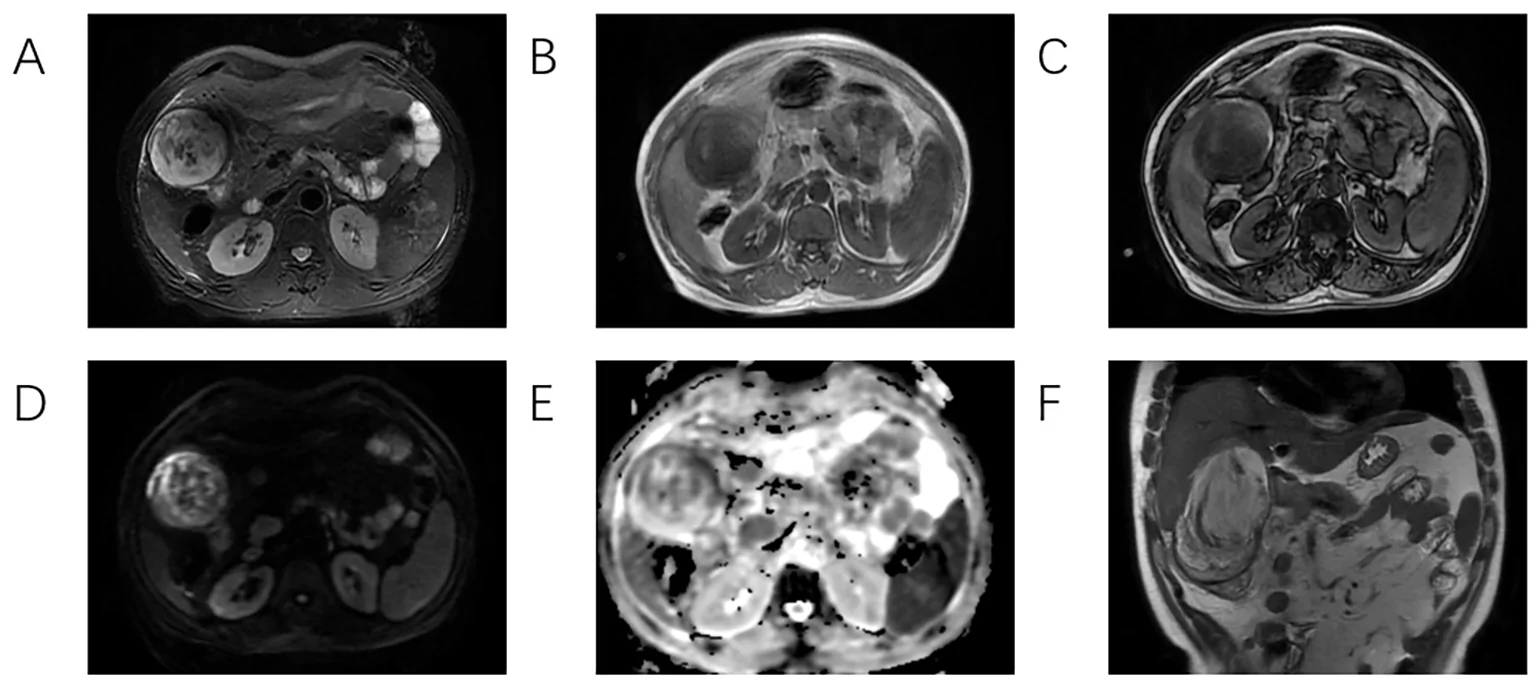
(A) T2WI: The gallbladder cavity exhibits mixed signal intensity with patchy isointense to hypointense areas of irregular morphology. (B) T1WI: The lesion demonstrates isointense to hypointense signal. (C) T1WI (reverse phase): No signal reduction observed in the lesion. (D) DWI: Patchy and punctate hyperintense signals are visible within the gallbladder lumen. (E) ADC: Signal reduction in the lesion. (F) T1W1 coronal view: Indicates tumour invasion into the hepatic flexure of the colon.
